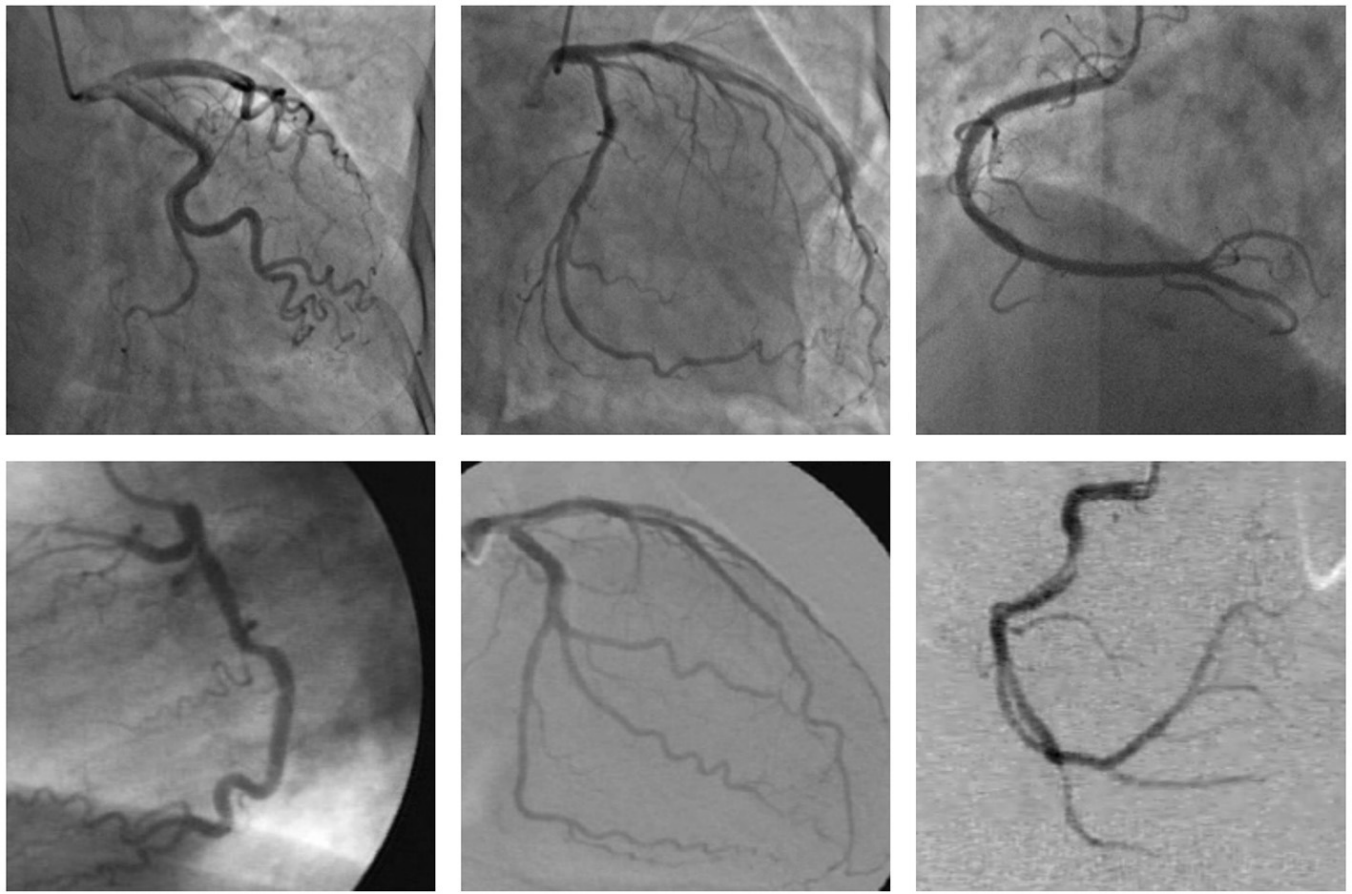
These examples of the X-ray angiogram image with nonuniform illumination, low contrast ratios, and low signal-to-noise ratios. The images in the first row are selected from the clinical coronary angiograms dataset, while the second row of the data is from the Database X-ray Coronary Angiograms.
